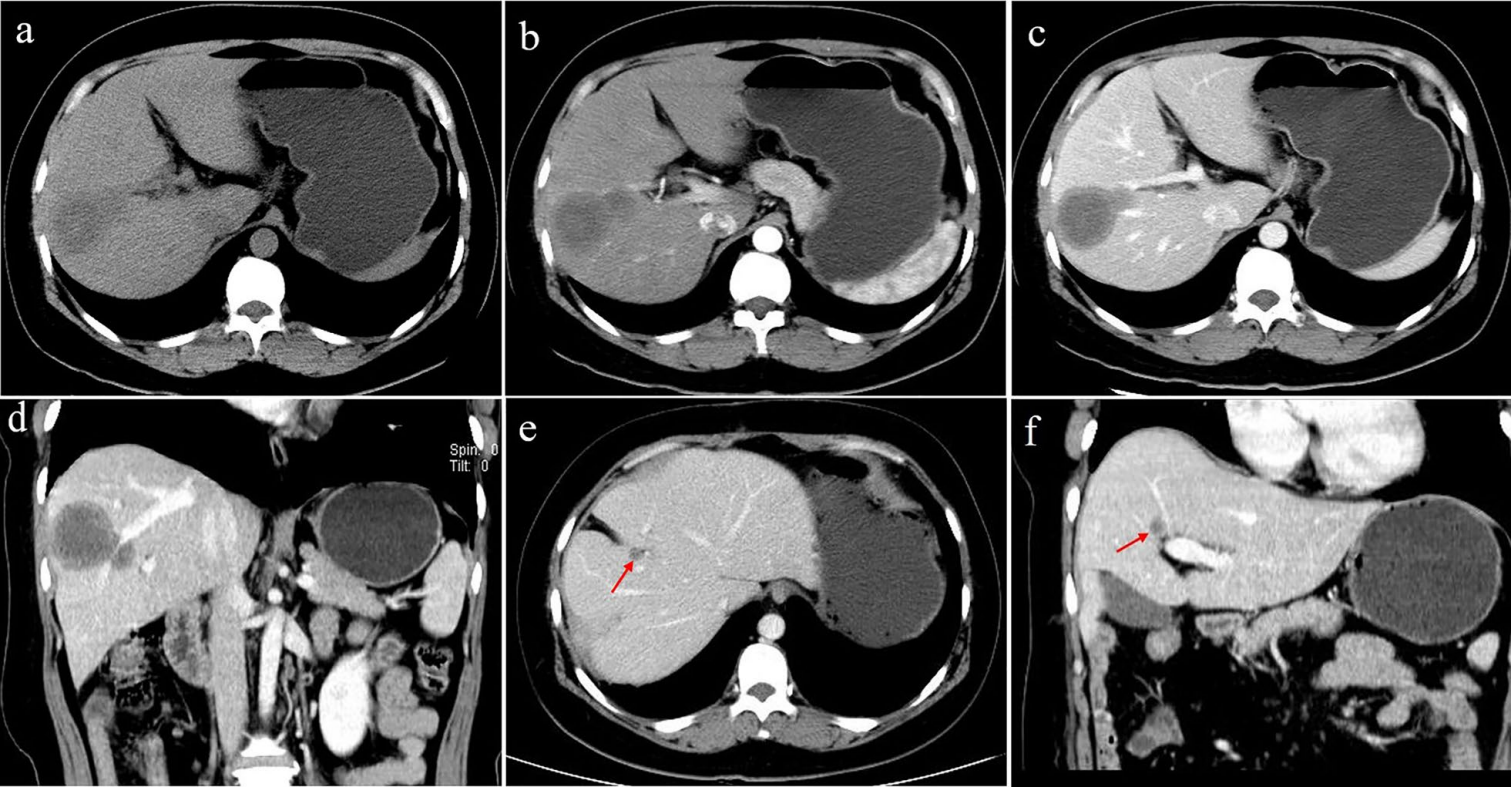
A 41-year-old female with solitary nodular type HEH. An axial unenhanced CT image a shows a round nodule with ill-defined, heterogeneous hypodensity. An axial arterial phase image (b) shows rink-like enhancement. An axial portal phase image (c) shows “target” sign enhancement. Coronal reconstruction of the portal venous phase (d) shows a narrowing portal vein branch adjacent to the lesion. This patient was initially misdiagnosed with hepatic abscesses. On follow-up CT performed 10 months after resection (e, f), a new lesion was detected in the right lobe of the liver (red arrowheads)
